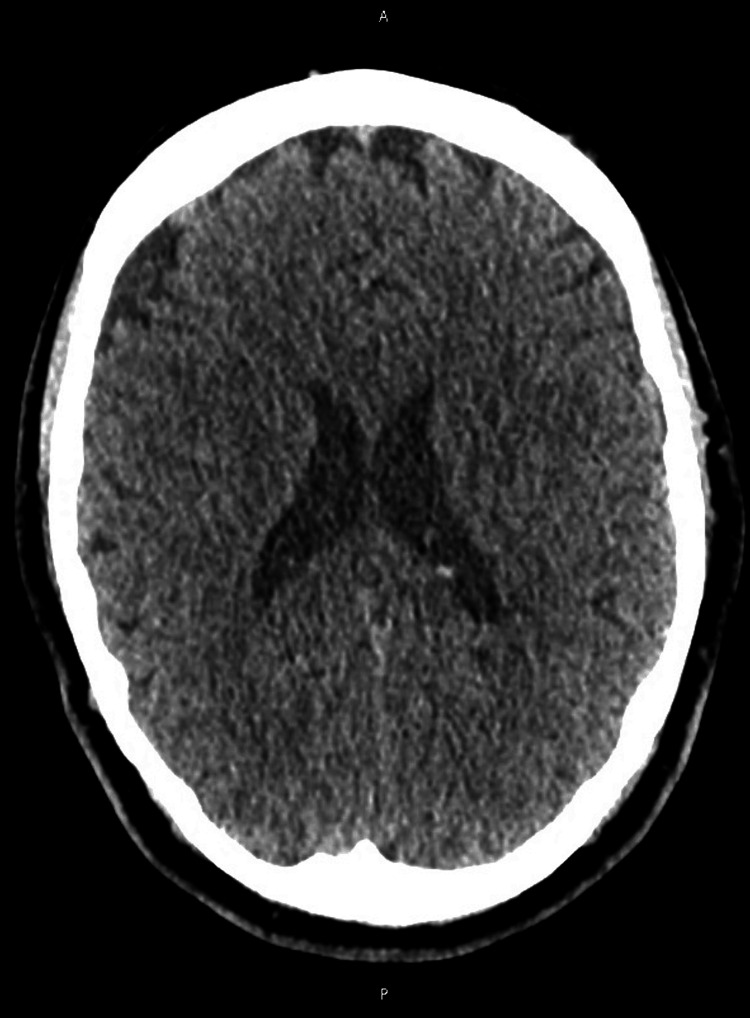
Computed tomography (CT) image of the brain No evidence of acute intracranial abnormality is visible.
